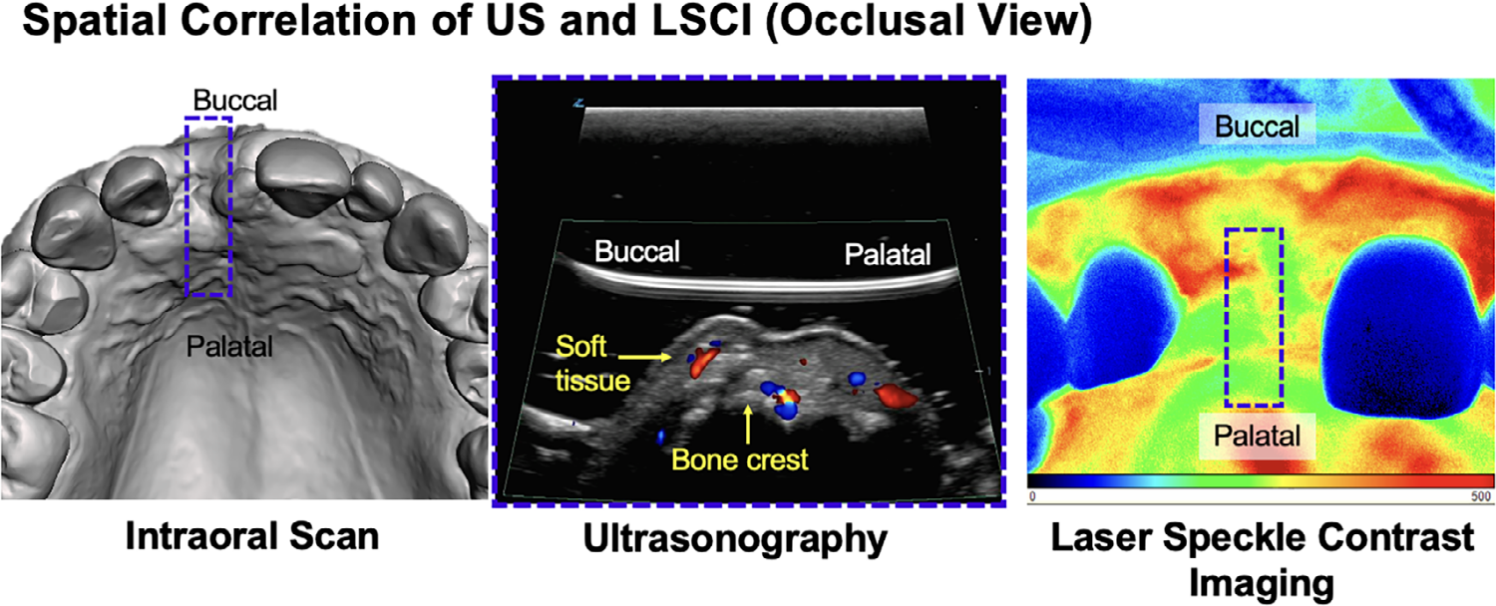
Spatial correlation of the edentulous ridge in anterior maxillary site (#8) using ultrasound (US) and laser speckle contrast imaging (LSCI) in an occlusal view. This figure demonstrates the complimentary benefits of combing the two techniques for evaluation of blood perfusion because US and LSCI offer cross‐sectional/deep‐perfusion and projection/superficial‒perfusion views, respectively.
